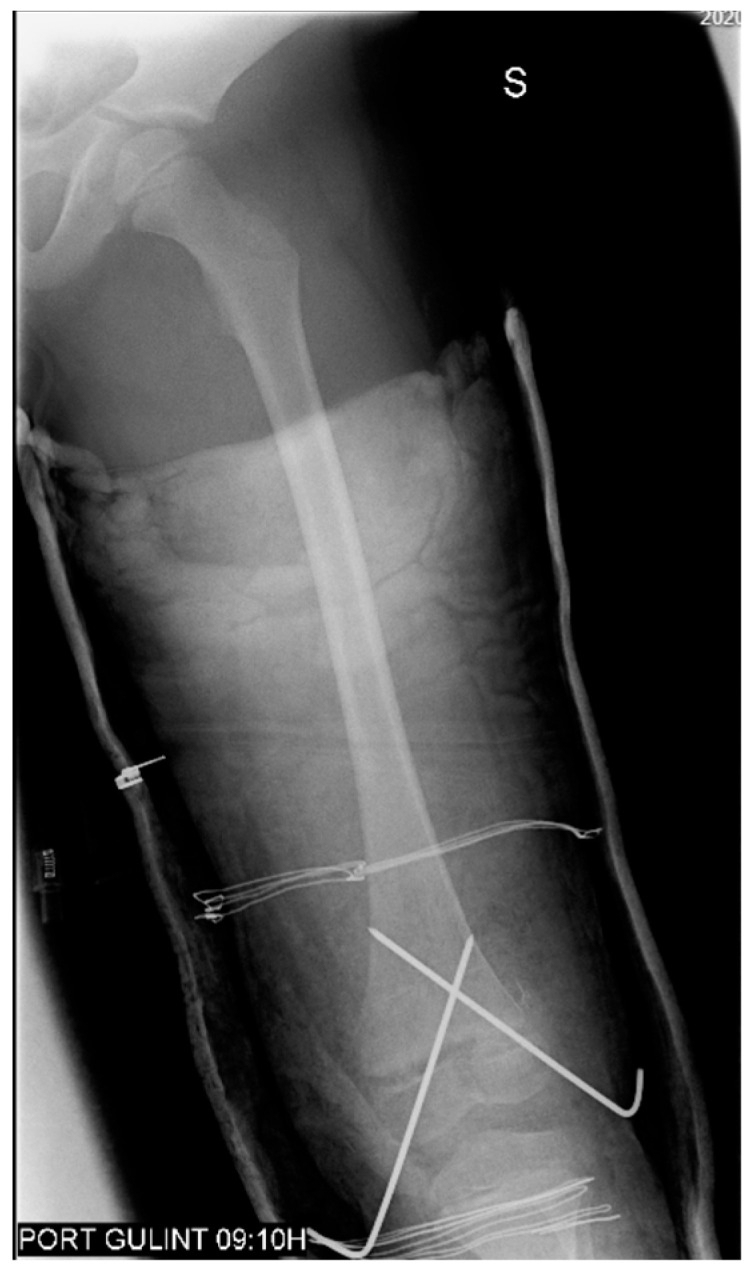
Postoperative anteroposterior radiograph demonstrating crossed smooth Kirschner-wire fixation of the distal femoral physeal fracture in a cast mobilization.
